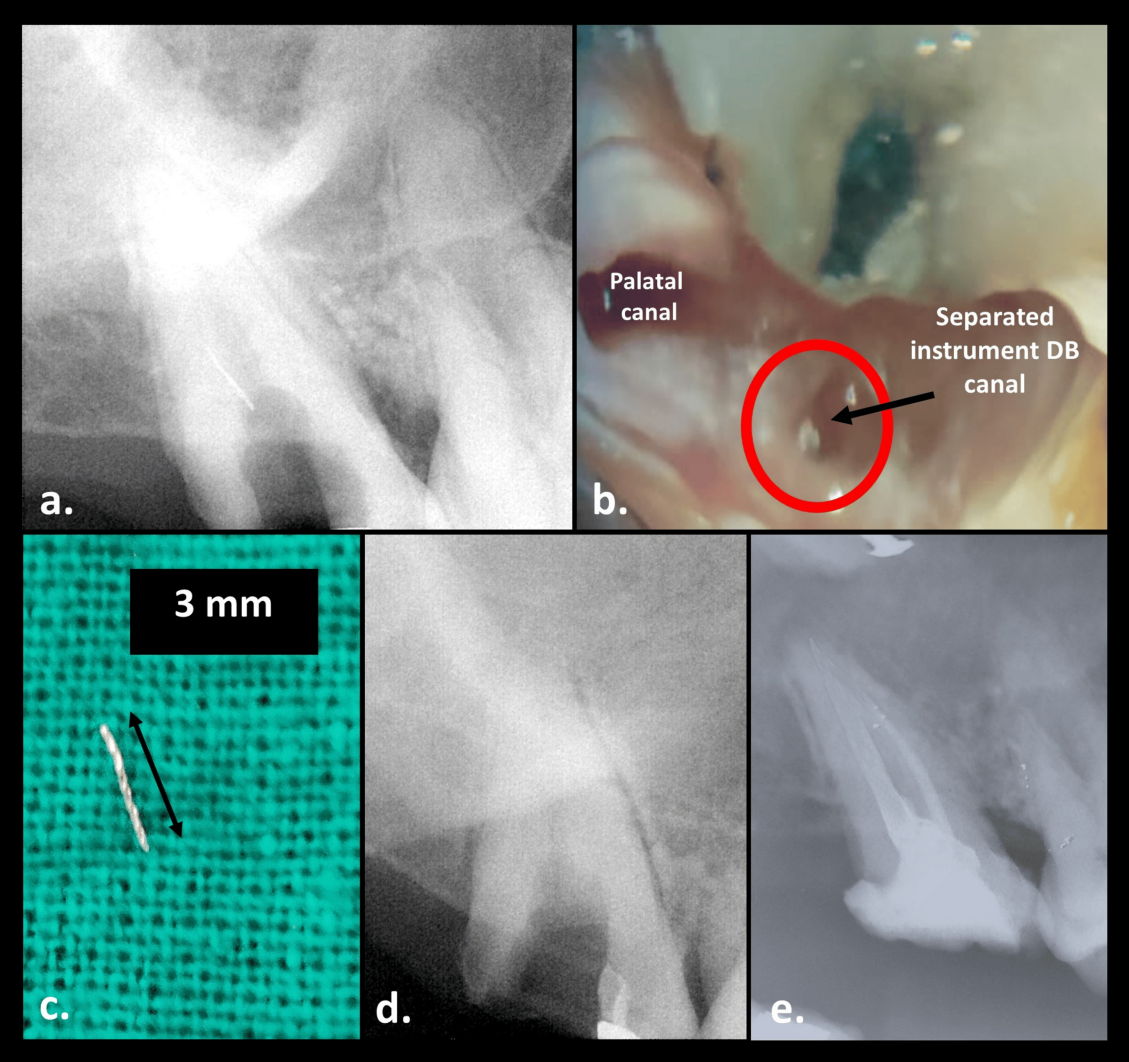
Non-surgical instrument retrieval: (a) preoperative radiograph, (b) locating separated fractured fragment (30×), (c) retrieved instrument, (d) confirmatory radiograph, and (e) post-obturation radiograph
